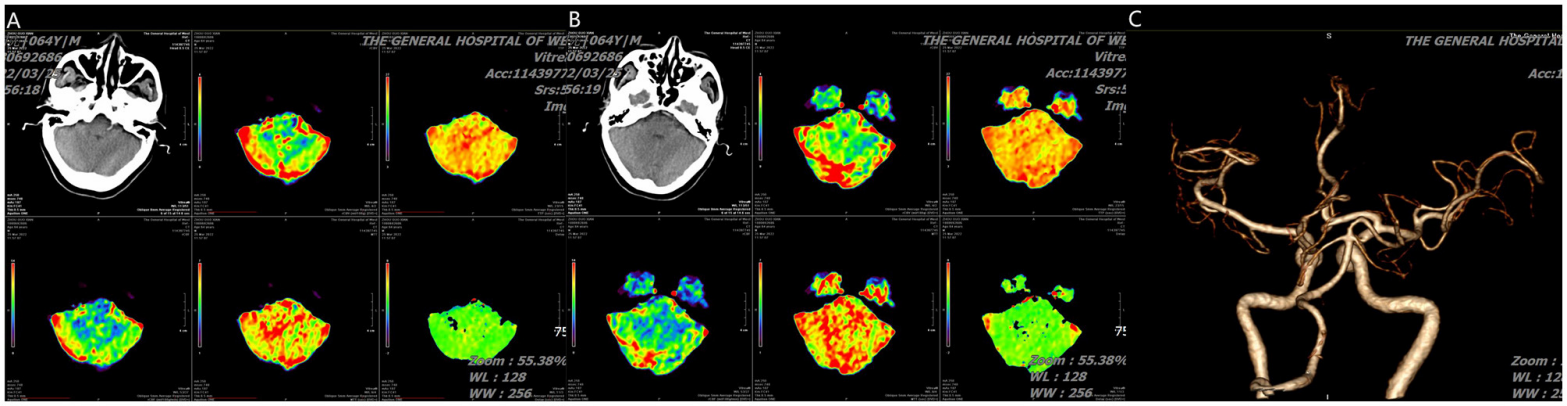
(A, B) CT perfusion show cerebellar infarction with small core infarction but large ischemic penumbra and (C) angiography (posterior-anterior view) show absence of right vertebral artery and normal basilar artery.
